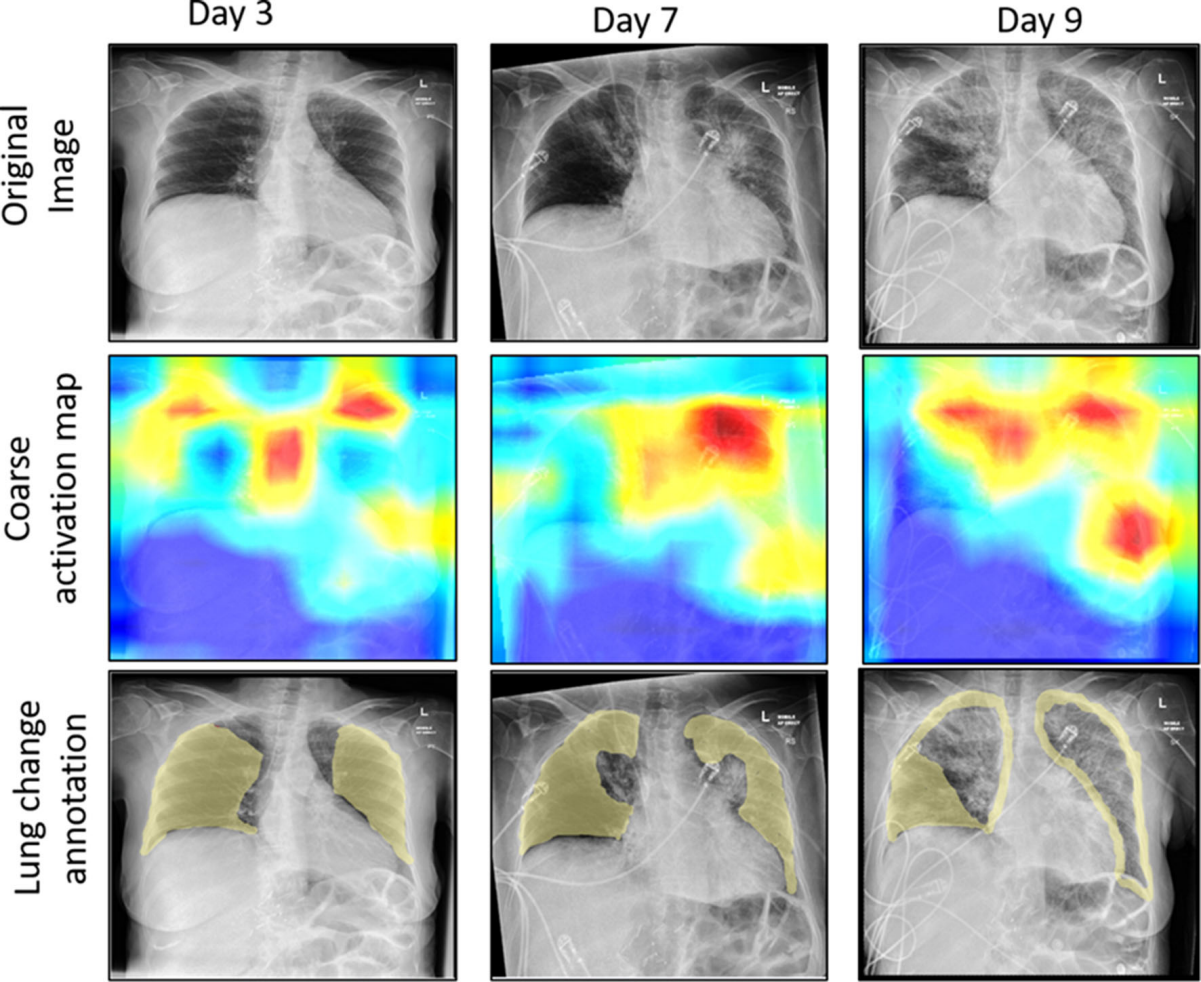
Coarse activation map visualization for a patient’s X-ray in ICU-care at day 3, 7 and 9, visualising increased inflammation (e.g. consolidations and ground-glass opacity) indicating disease severity
